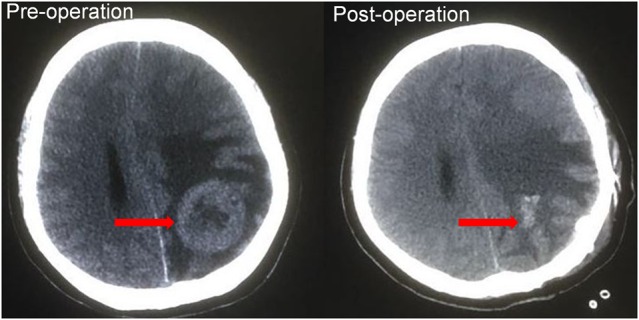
Cranial computerized tomography (CT) CT revealed the brain metastatic lesion and brain midline shift. There is a slightly high density nodule in the left parietal lobe, and the surrounding is the low density edema before operation, and patchy opacity left there after operation.
